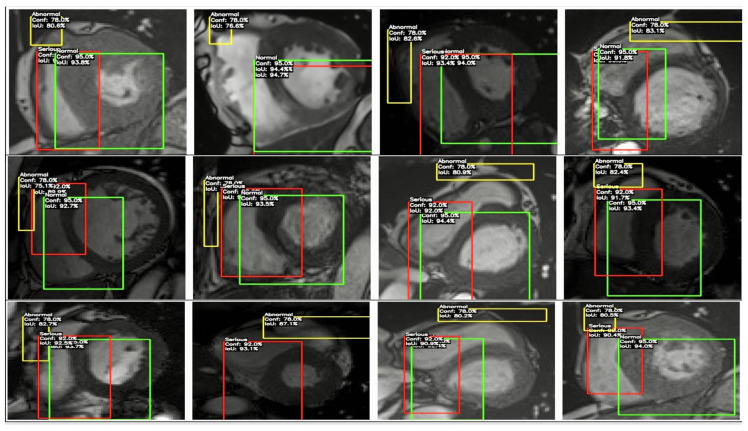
This figure depicts the YOLO model’s classification and bounding box detections on cardiac MRI images, identifying regions as ‘Normal’ (green), ‘Serious’ (red), and ‘Abnormal’ (yellow), along with confidence scores and IoU values. This visualization highlights detection consistency across severity levels, providing insights into potential algorithmic biases in cardiovascular imaging and supporting the goal of fairer, more reliable diagnostics.
